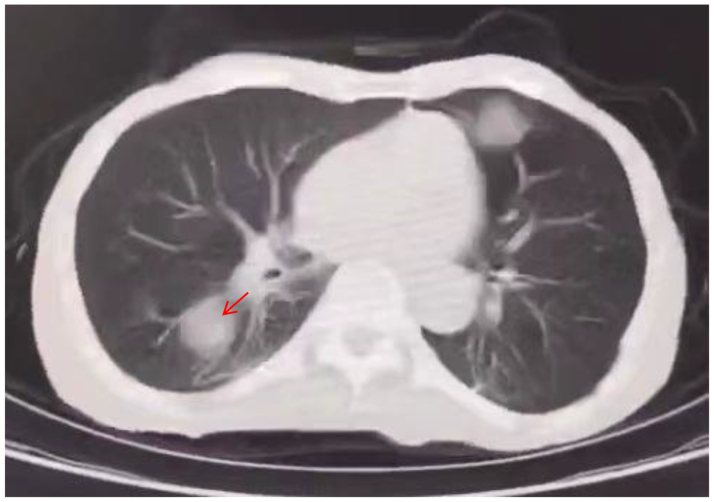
Follow-up chest CT shows lung metastasis. CT = computed tomography.
